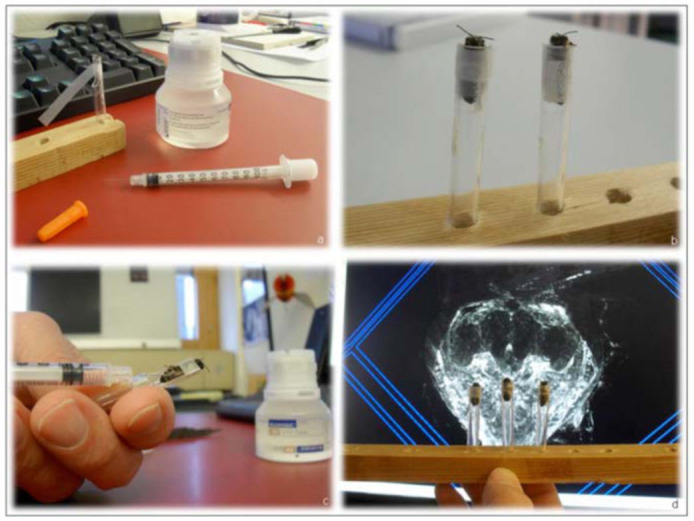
To enhance brain tissue differentiation, bolus injections of radiographic contrast media were delivered via a 3OG needle (a) directly into the haemolymph, between the dorsal abdominal terga, of live bees that were previously secured for scanning (b and c). The 3D rendered brain (d) showed that contrast had perfused into tissue to enable improved structural differentiation. High quality figures are available online.
